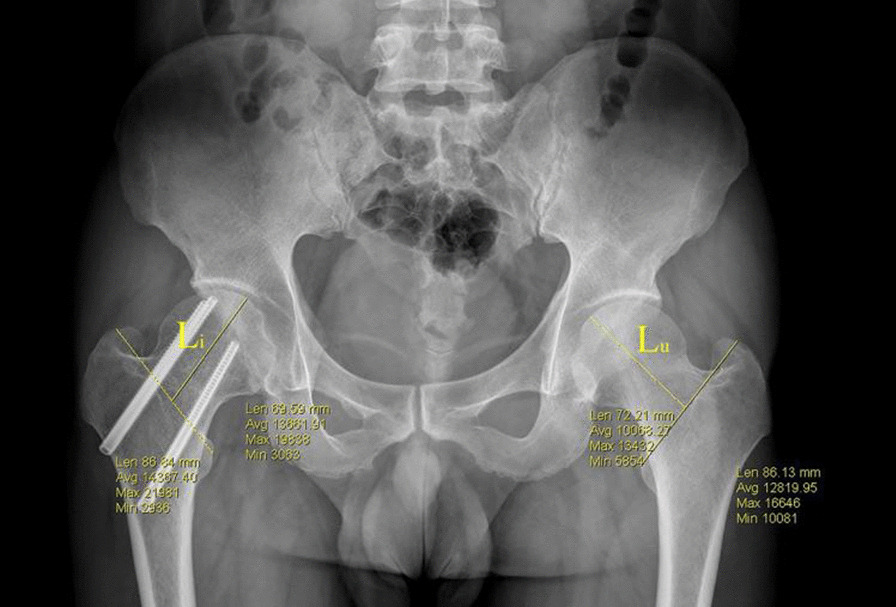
The measurement of femoral neck shortening. On the anteroposterior radiograph, the line was made through the center of the femoral head in the long axis of the femoral neck on both sides. The distance between the tip of the femoral head and the intertrochanteric line was measured. And the length of femoral neck shortening is the distance on the uninjured side minus the distance on the injured side
